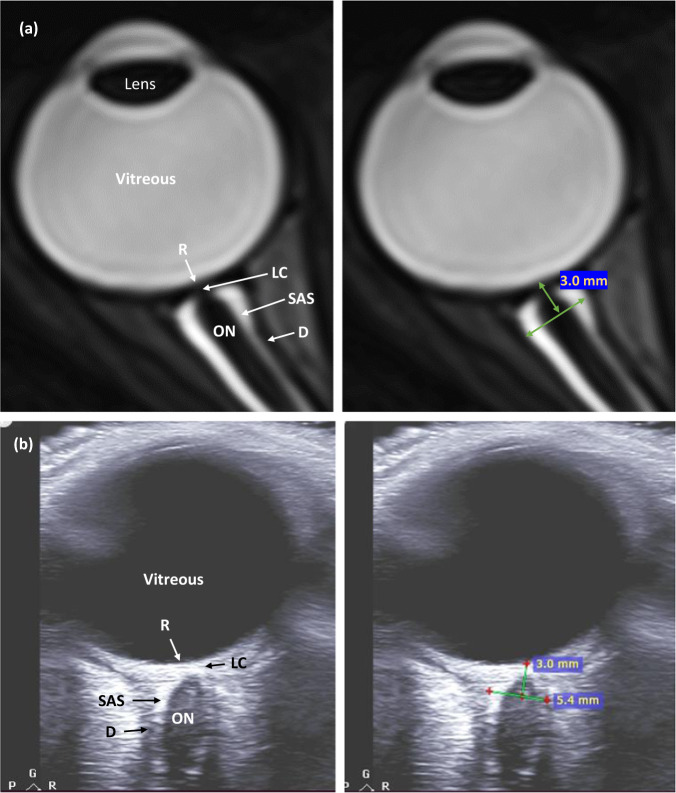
Example of anatomy and correct measurement of ONSD. Both MRI (a) and US (b) are represented in the figure. Note the measurement was conducted on a plane where the hypoechoic arachnoid and dural layer are clearly distinguished from the hyperechoic retroorbital face and subarachnoid space. R Retina, LC lamina cribrosa, ON optic nerve, SAS subarachnoid space, D dura.
